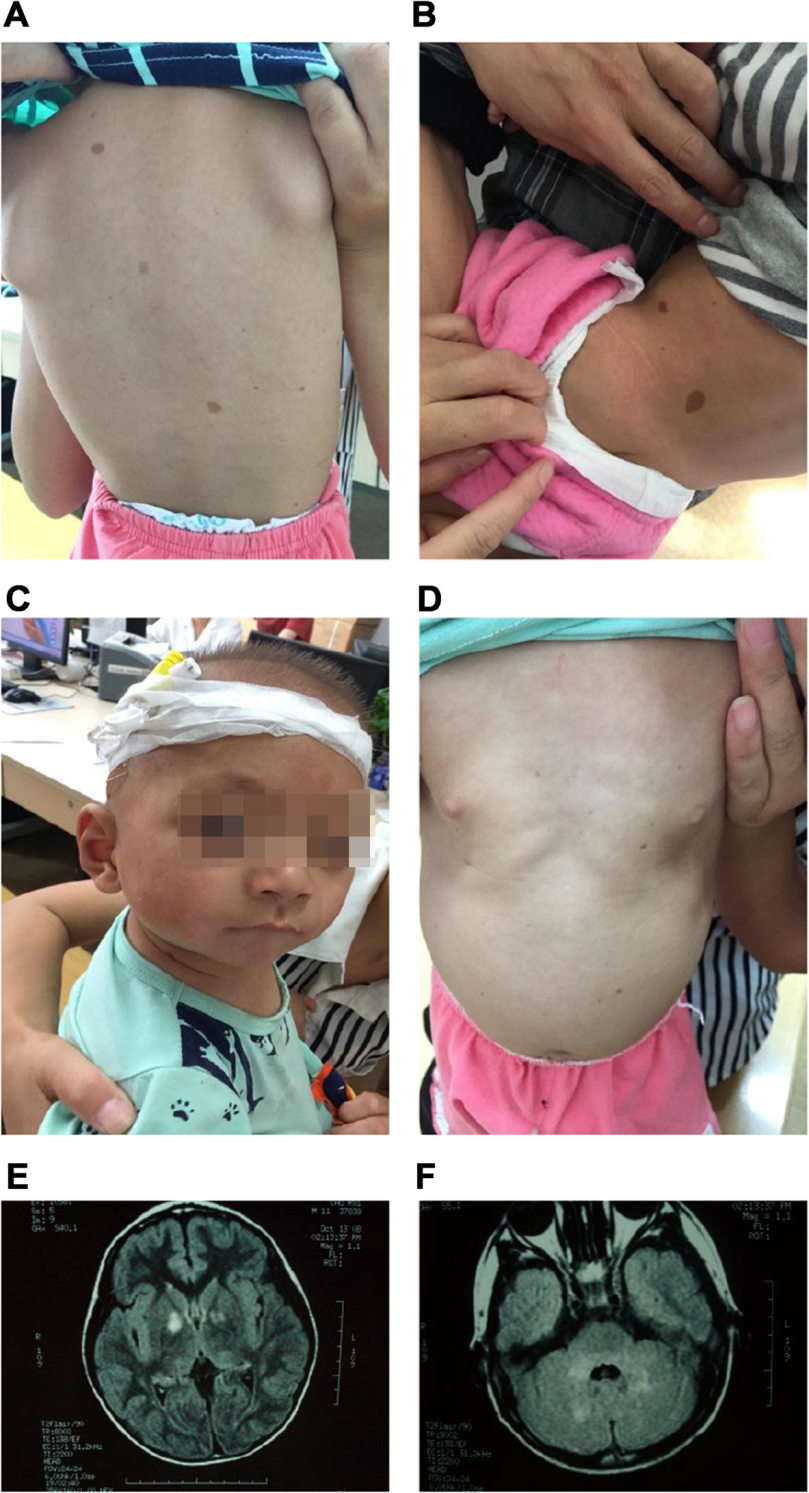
a-b Café-au-lait spots on the skin. c Unusual face, with widely-spaced eyes and left blepharoptosis. d Pectus excavatum. e-f Cranial MRI showing lesions with high signal intensity on T2FLAIR in basal ganglia and cerebellum
